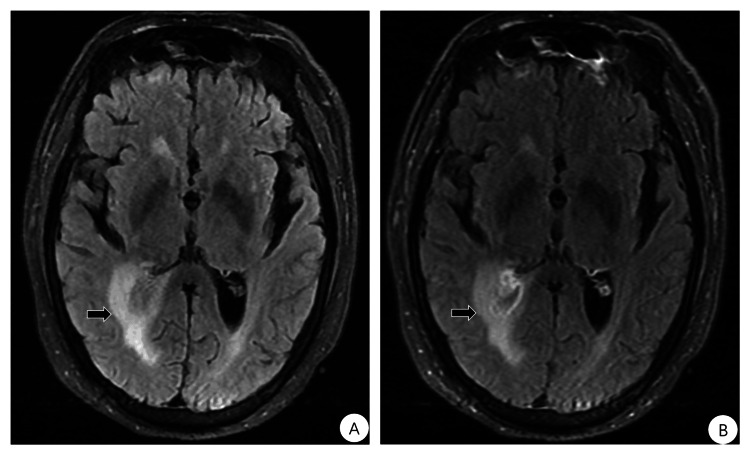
Follow-up brain MRI after one week of treatment with cefotaxime 2g every 12 hours and methylprednisolone 20mg/day indicated a slight enlargement of the lesion. T2/FLAIR (A) and enhanced FLAIR (B).
